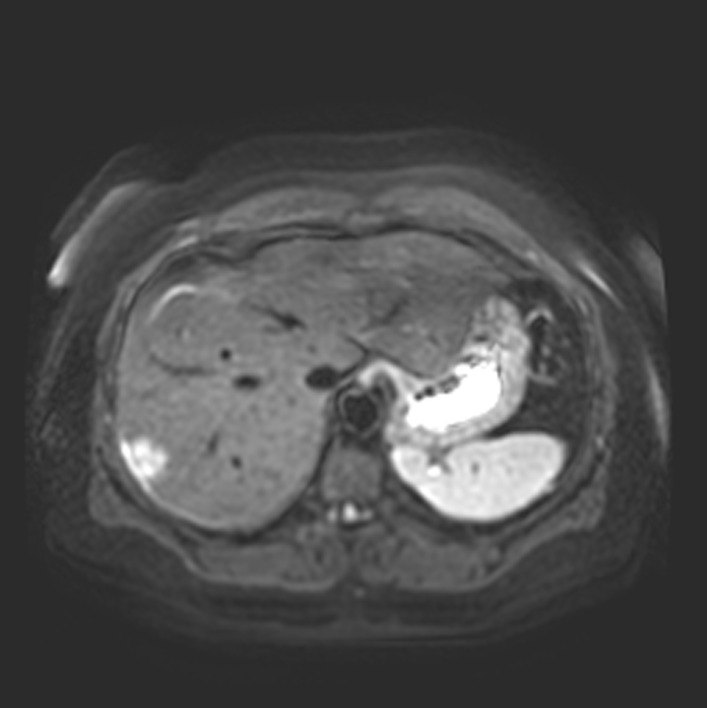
Initial abdominal MRI. In the periphery of the right hepatic lobe there is a somewhat ill-defined lobulated region of T2 hyperintensity about 2.3 cm in size. Centrally, the signal is similar to the hepatic parenchyma. This area has heterogeneous restricted diffusion
